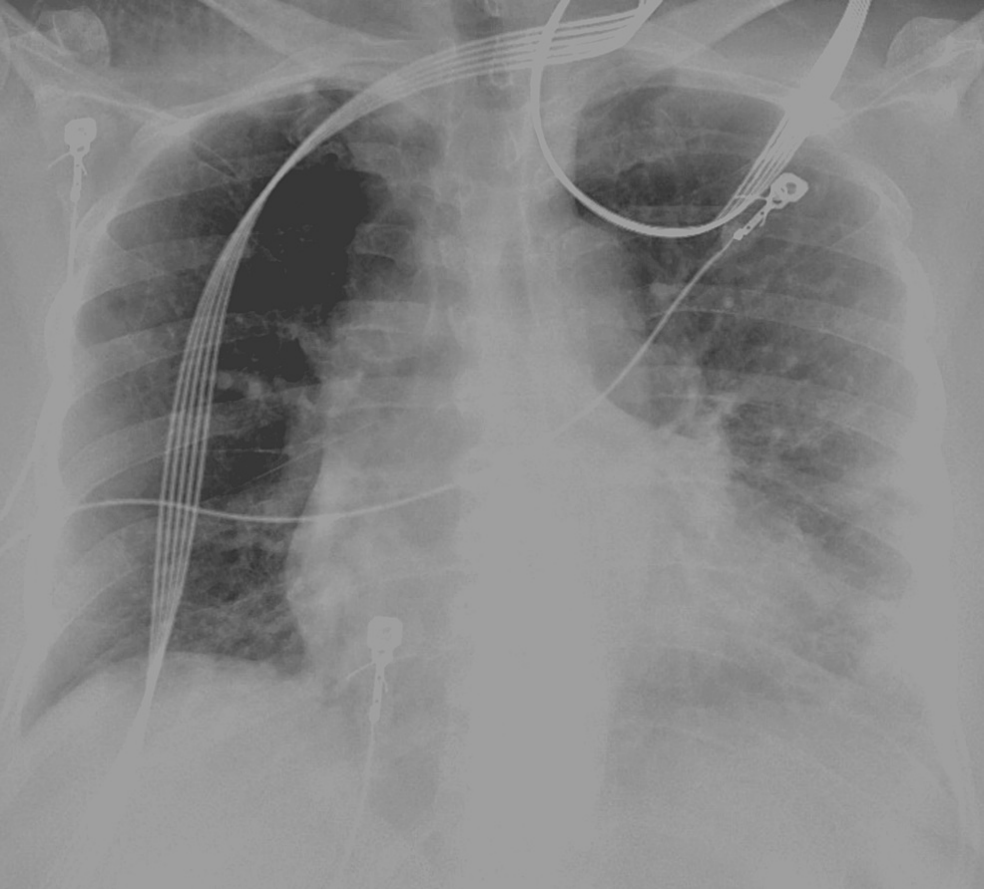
Chest x-ray on admission showing patchy lower left lung infiltrates compatible with pneumonia
